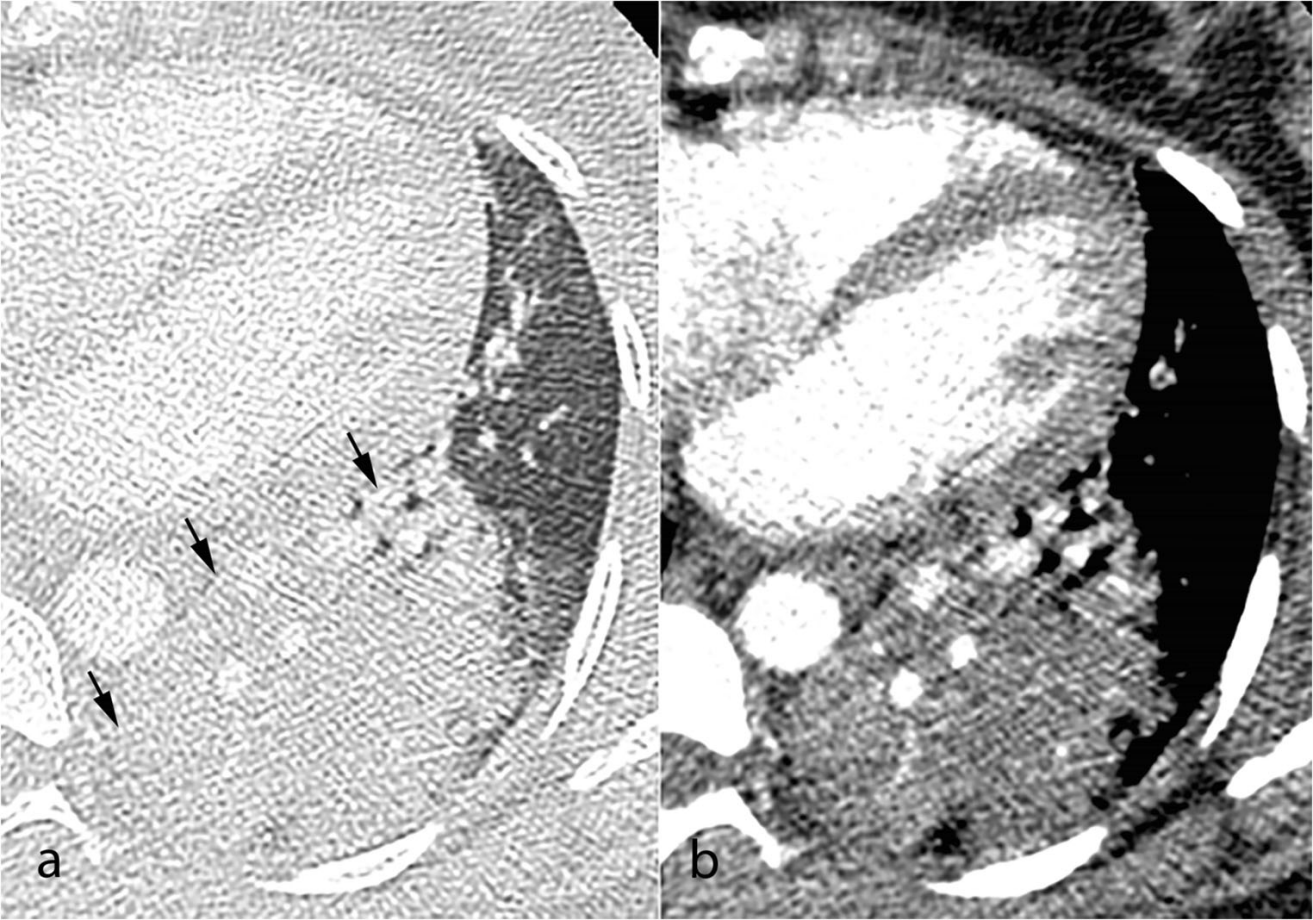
Axial computed tomography pulmonary angiography images in the lung (a) and the soft tissue windows (b), from a 33-year-old pregnant patient presenting with acute chest pain and dyspnea. No pulmonary emboli were detected; however, the scan revealed left lower lobe consolidation consistent with pulmonary infection (a, black arrows)
